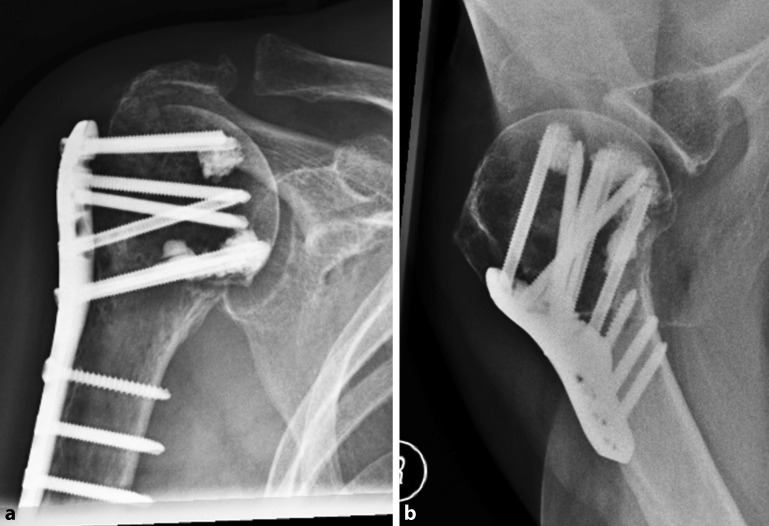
Radiographs 3 months after locked plating of a proximal humeral fracture (see Fig. 1) with additional cement augmentation of the anterosuperior and inferior humeral head screws showing fracture union without implant related complications (a anteroposterior, b axillary view)
